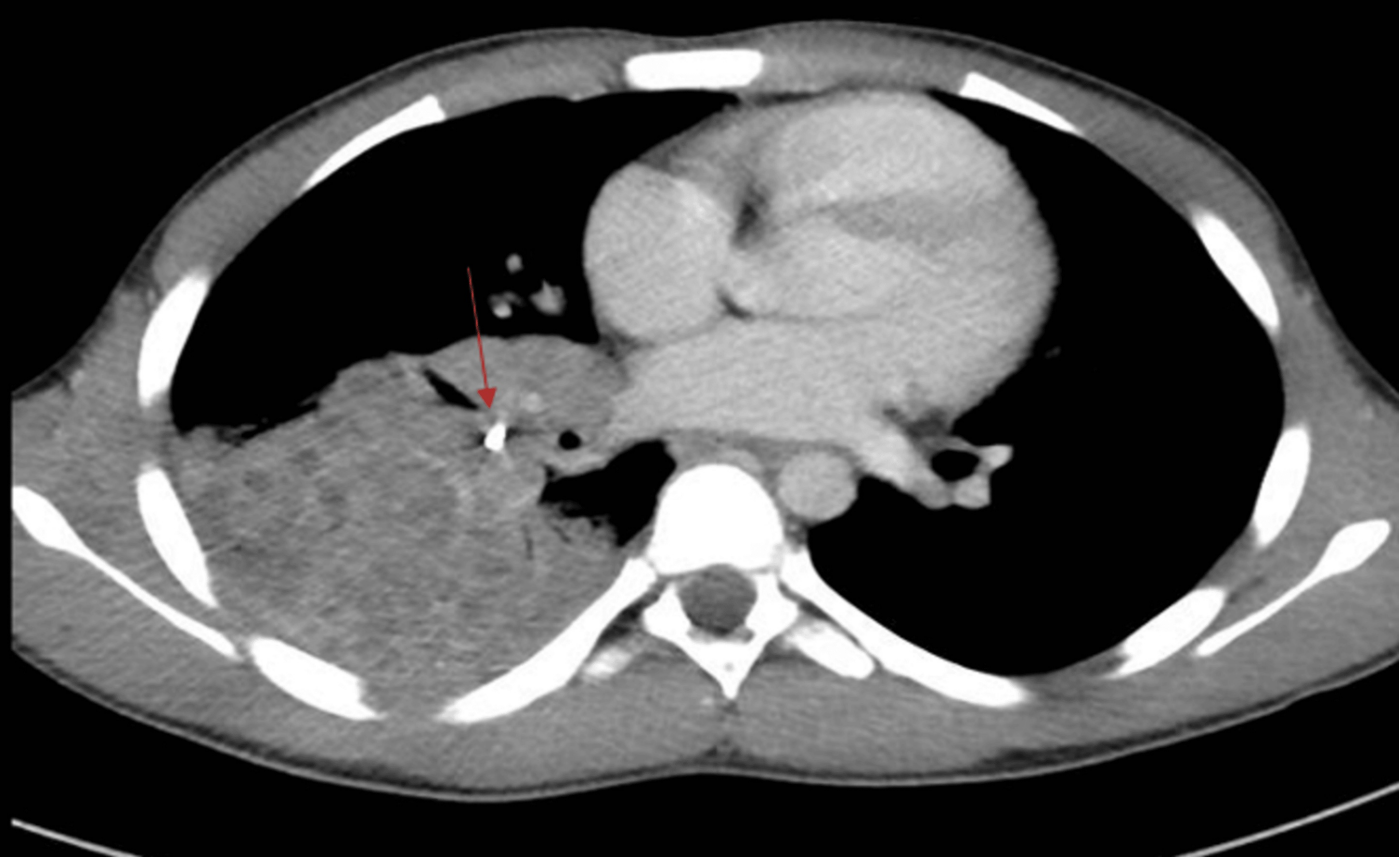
CT chest revealing the foreign body in the bronchus intermedius and right lower lobe bronchiectasis CT: computed tomography
